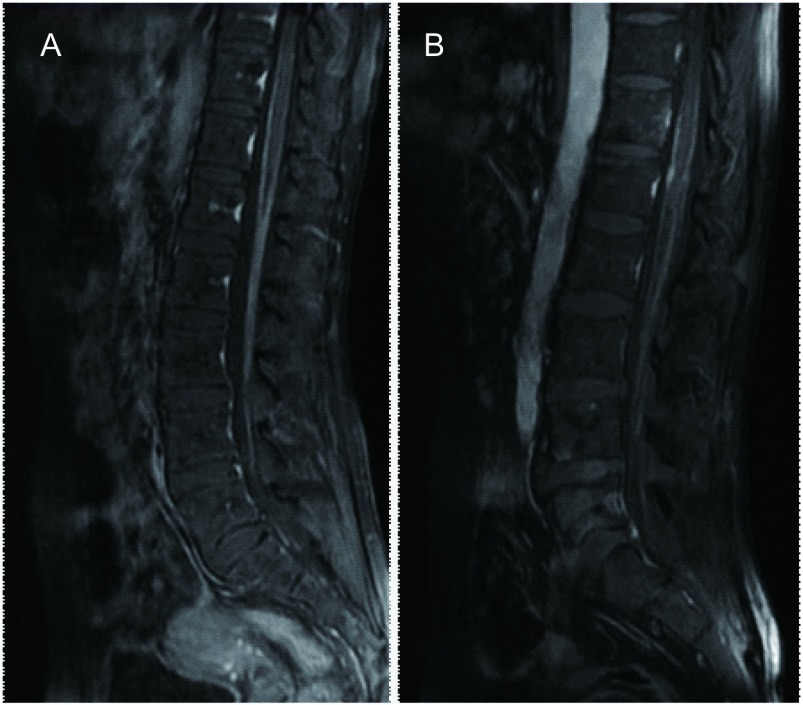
No.3(A)及No.2(B)患者T1压脂腰椎增强MRI表现。腰椎增强MRI显示软脊膜不均匀增厚伴有线样强化。 Sagittal sections of a contrast-enhanced fat-saturated T1-weighted magnetic resonance imaging (MRI) study of the lumbar spinal cord of patient No.2 (B) and patient No.3 (A).Contrast-enhanced MRI of lumbar spine showed diffuse abnormal enhancement of pial lining of spinal cord.
